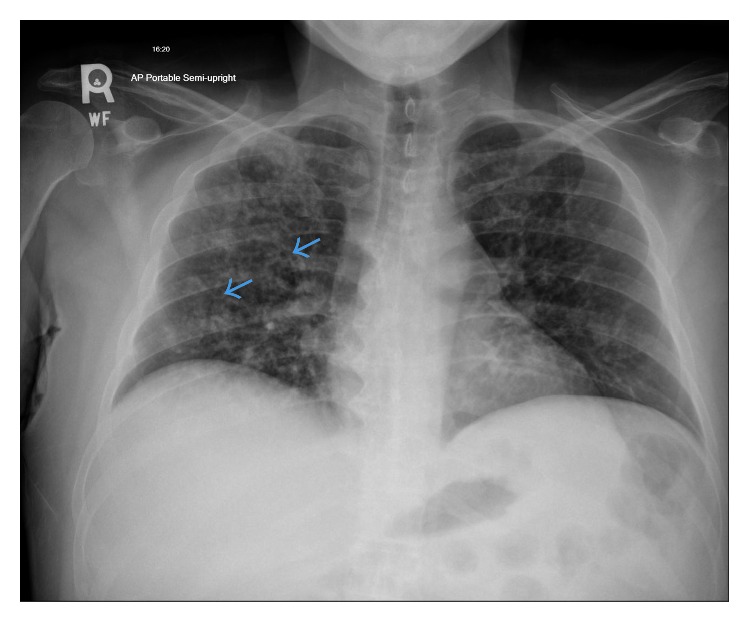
Chest roentgenogram at referring hospital. AP chest radiograph at outside hospital performed 4 days prior. Diffuse micronodules greater in the right lung are seen. Hint of small cavities is noted (blue arrows).
